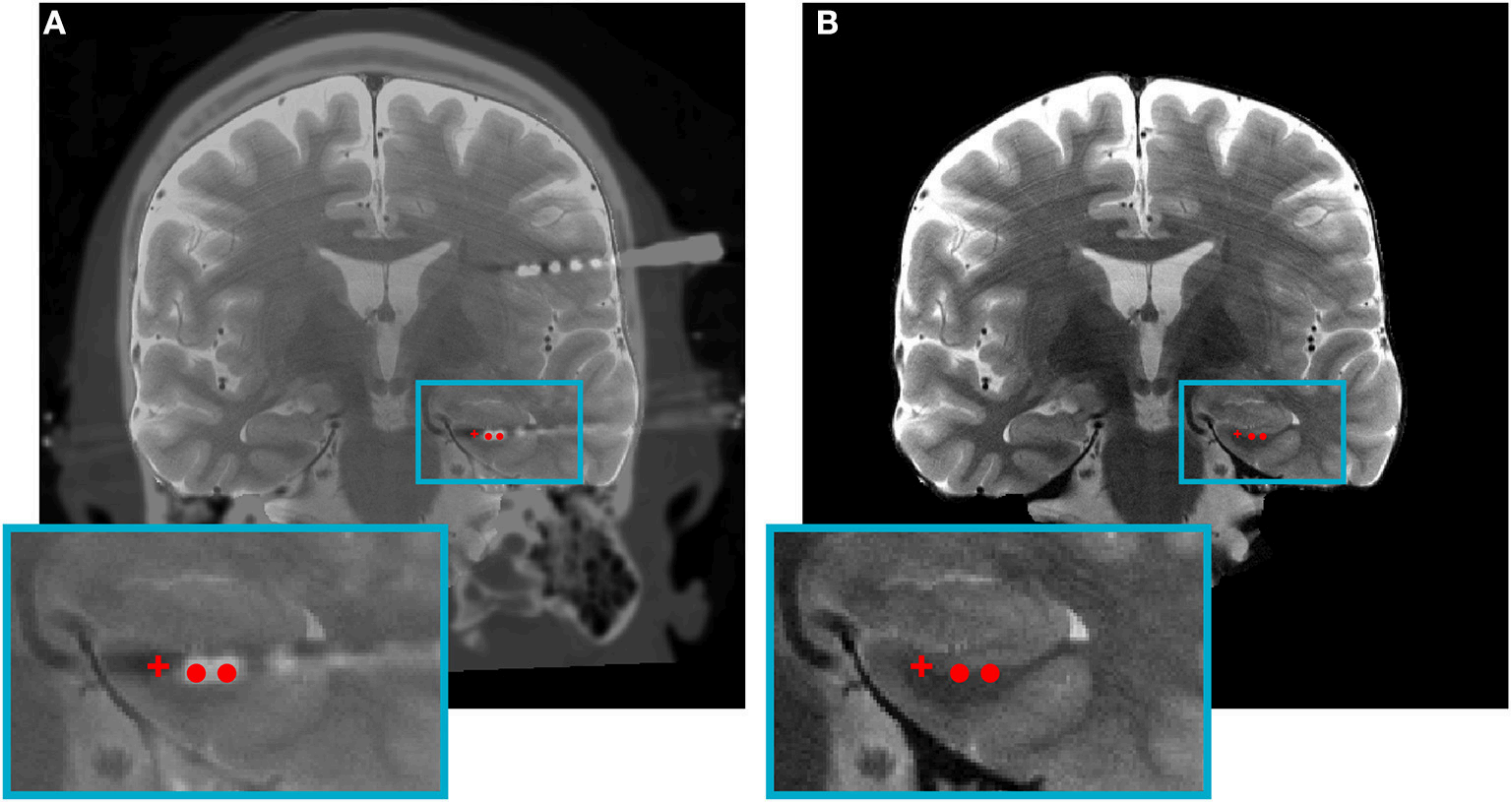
An example image for studies to follow when reporting single participant electrode localizations for bipolar macro-stimulation or monopolar micro-stimulation. (A) Coronal view of a co-registered image of a pre-implantation high-resolution CT and post-implantation high-resolution MRI with overlaid electrode contact segmentations. (B) The outcome of the electrode localization procedure where individual electrode contact locations within the left hippocampus are overlaid onto a high-resolution MRI for final visualization and reporting. Red circles: bipolar macro-stimulation contacts; red crosshair: monopolar microstimulation contact.
